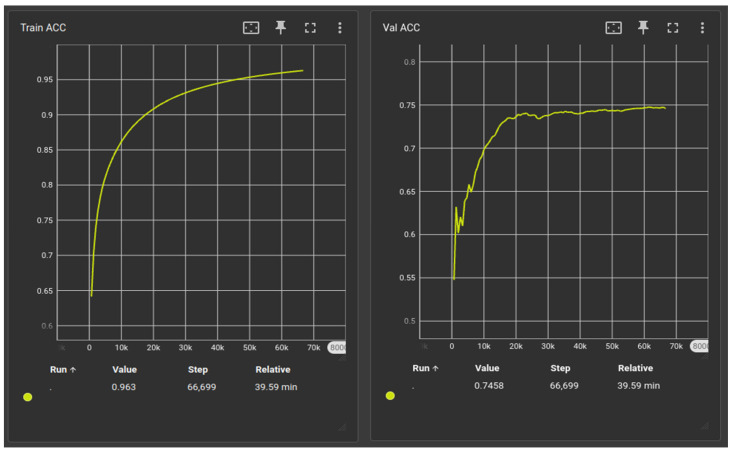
Training and validation accuracy during the training of the CT-based model.
